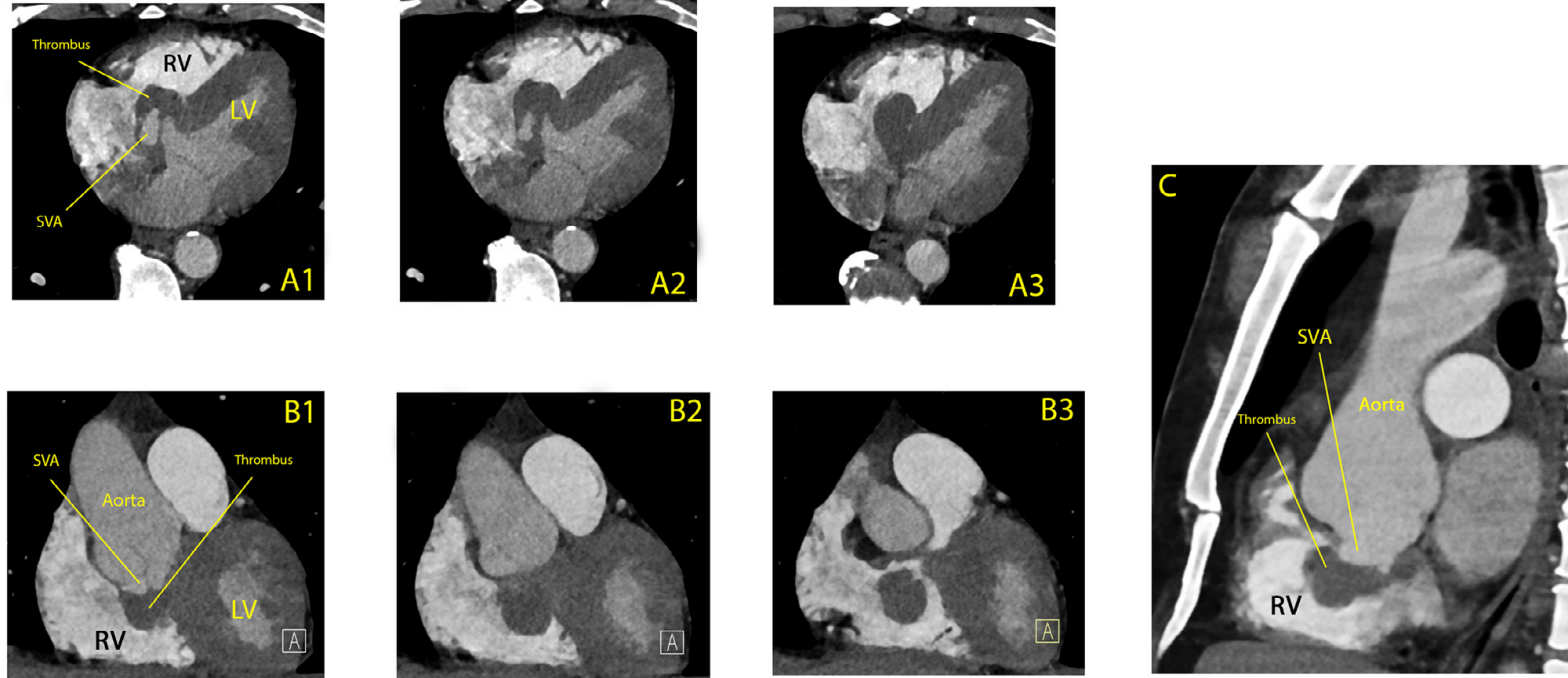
Cardiac Computed Tomography Angiography scan with images of a Sinus Valsalva Aneurysm (SVA) of the non-coronary cusp bulging into the right ventricle. Images are shown in axial (panel A1-A3), coronal (panel B1-B3) and sagittal (C) view. *RV: right ventricle, LV: left ventricle.*
